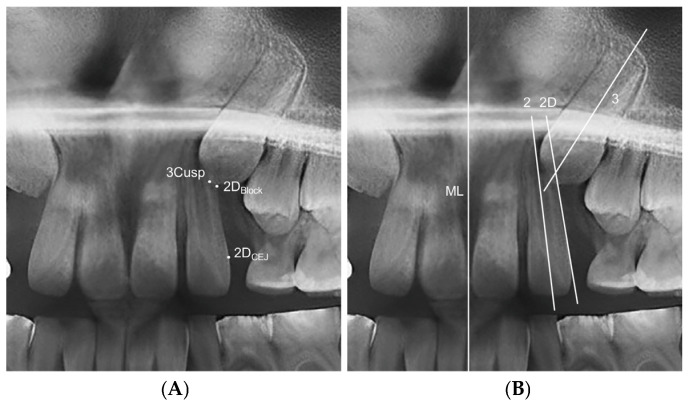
Panoramic radiographs showing: (A) reference points; (B) reference lines. Detailed descriptions of all reference points and lines are provided in Table 1.
